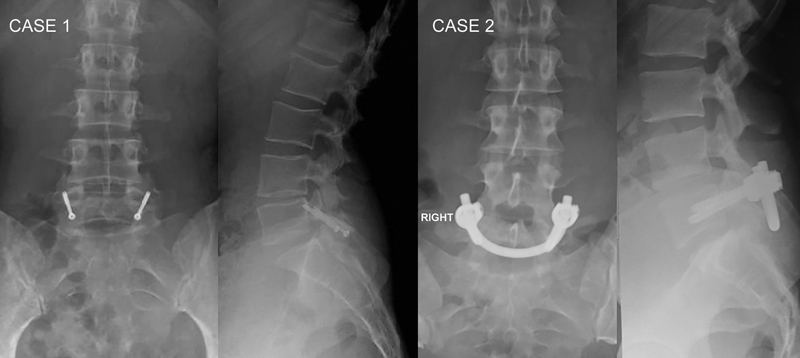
Postoperative radiographs. Case 1, modified Buck technique. Case 2, Smile technique.
